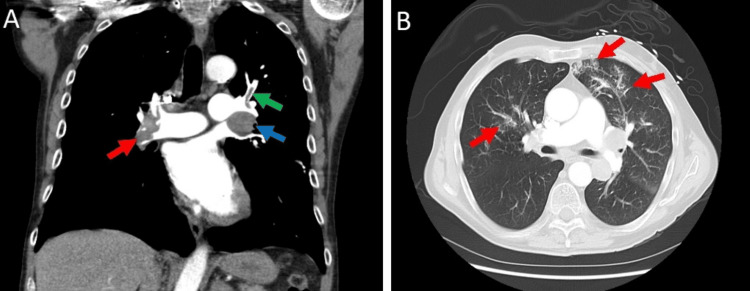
CTPE protocol of the chest. (A) Contrast-enhanced angiogram showing a large thrombus at the right main pulmonary artery (red arrow) and a thrombus on the left upper branch of the pulmonary artery (green arrow). There is also enlarged left hilar lymphadenopathy measuring up to 2.5 x 3.3 cm (blue arrow). (B) Contrast-enhanced angiogram showing ground glass opacities bilateral lungs (red arrow) suspicious of pulmonary infarcts or pulmonary hemorrhages. CTPE: computed tomography pulmonary embolism.
